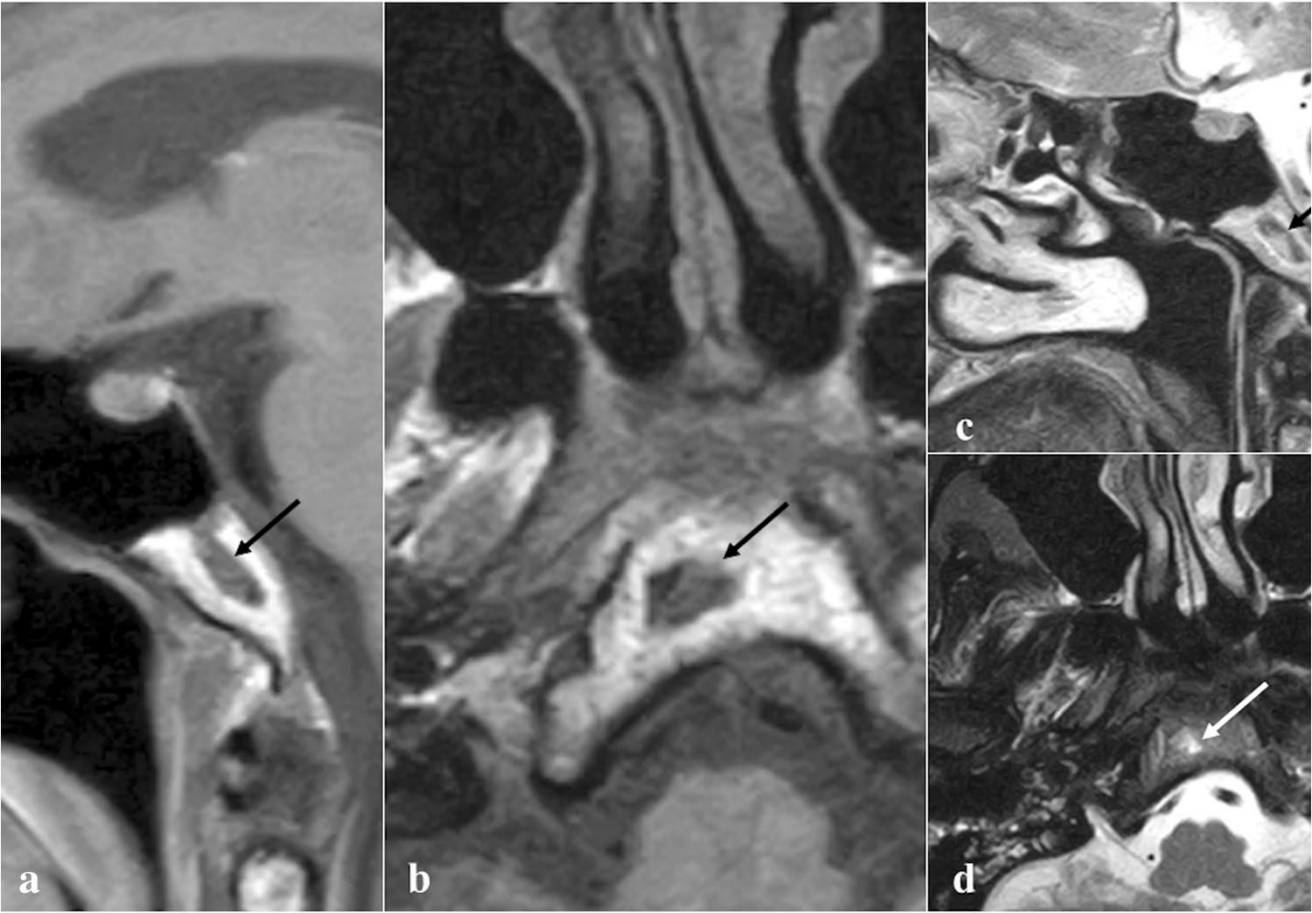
Neurenteric cyst of the clivus as an incidental finding in a 56-year-old female patient with headache. MRI shows an oval, intramedullary cystic lesion of the clivus (arrows). Compared to the cerebrospinal fluid, this lesion is characterised by intermediate-to-high SI on sagittal (a) and axial (b) T1W images, and high SI on sagittal (c) and axial (d) T2W images, thus reflecting high protein content
